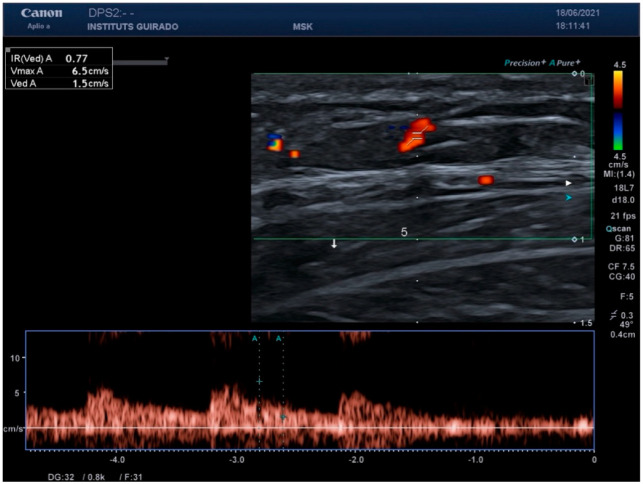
Spectral Doppler analysis—a spectral Doppler image depicting an arterial vessel in healthy skin, showcasing typical flow characteristics and peak systolic values.
